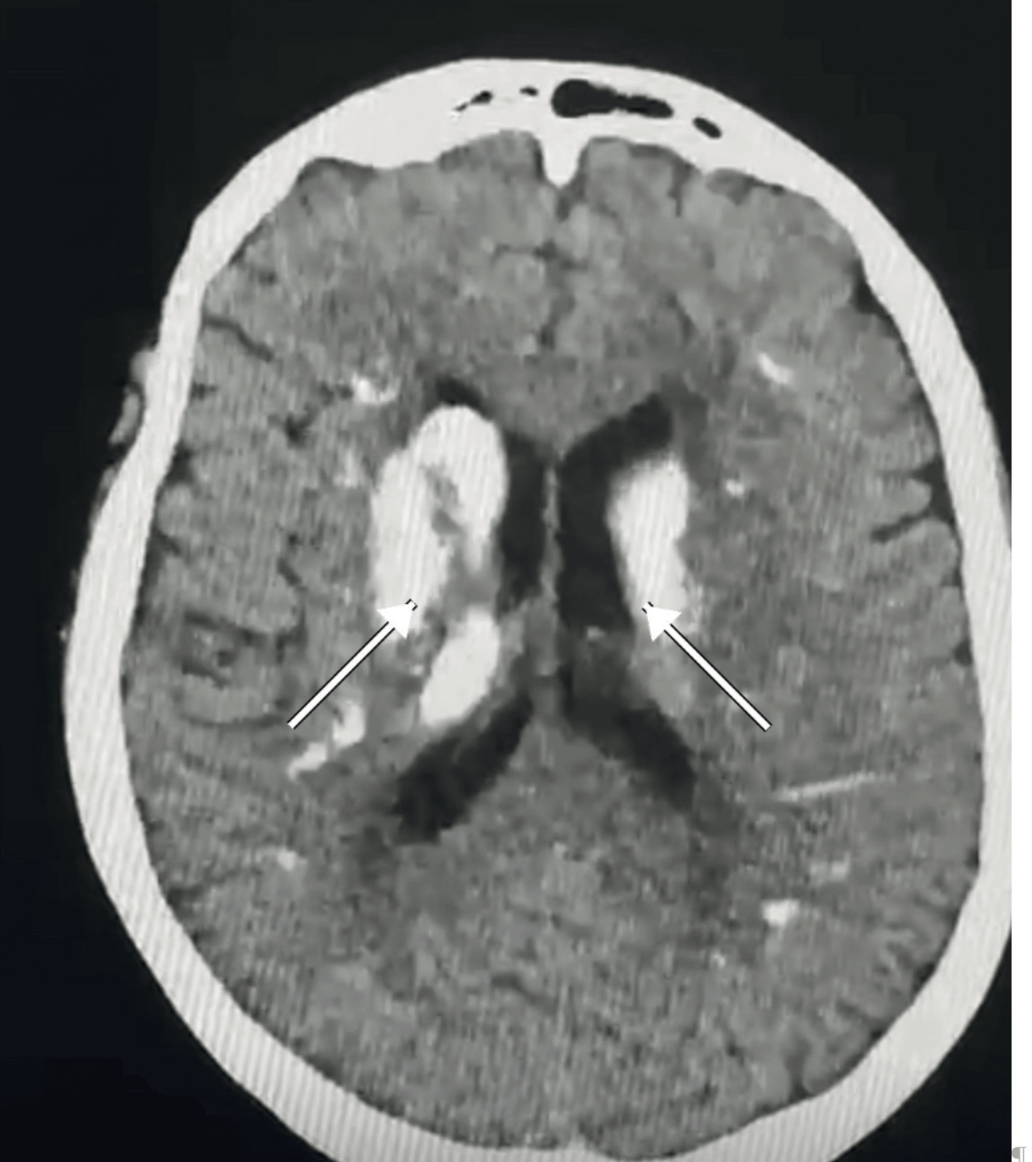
Axial non-contrast CT demonstrating bilateral, symmetric calcifications centered on the globus pallidus (arrows), consistent with Fahr syndrome secondary to hypoparathyroidism CT: computed tomography The figure is an original, de-identified CT image from the author’s clinical practice and has not been previously published.
